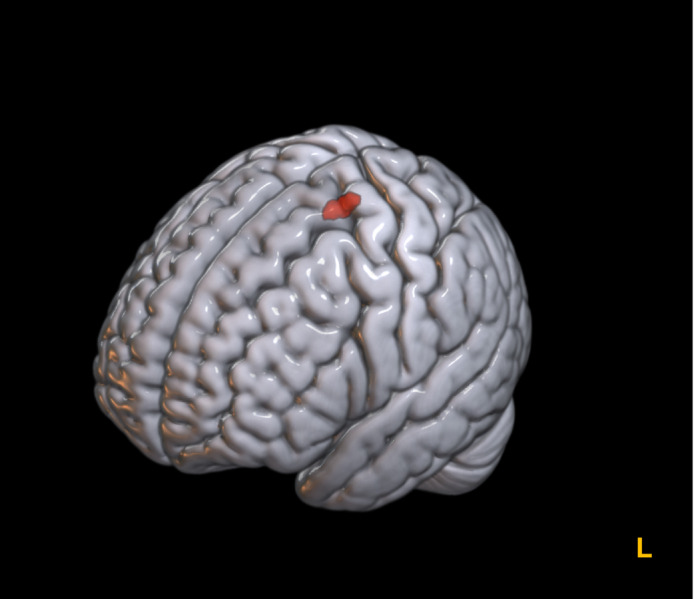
Convergent multimodal neurobiological changes in Treatment‐Resistant Depression (TRD). L, left. A significant convergence of neural differences estimated using a multimodal approach, including brain morphometry (gray matter volume) and resting‐state functional magnetic resonance imaging (low‐frequency fluctuations) in patients with TRD relative to healthy controls (HC) was found in a cluster in the left precentral/superior frontal gyrus (at *x*, *y*, *z* = [−12; −16; 62], here reported in red). The results are FWE corrected at 0.05 at the cluster level with cluster‐forming probability at *p* < 0.001 The probability map thresholded is rendered on a standard brain in Montreal Neurological Institute (MNI). The rendering was created using MricroGl.
